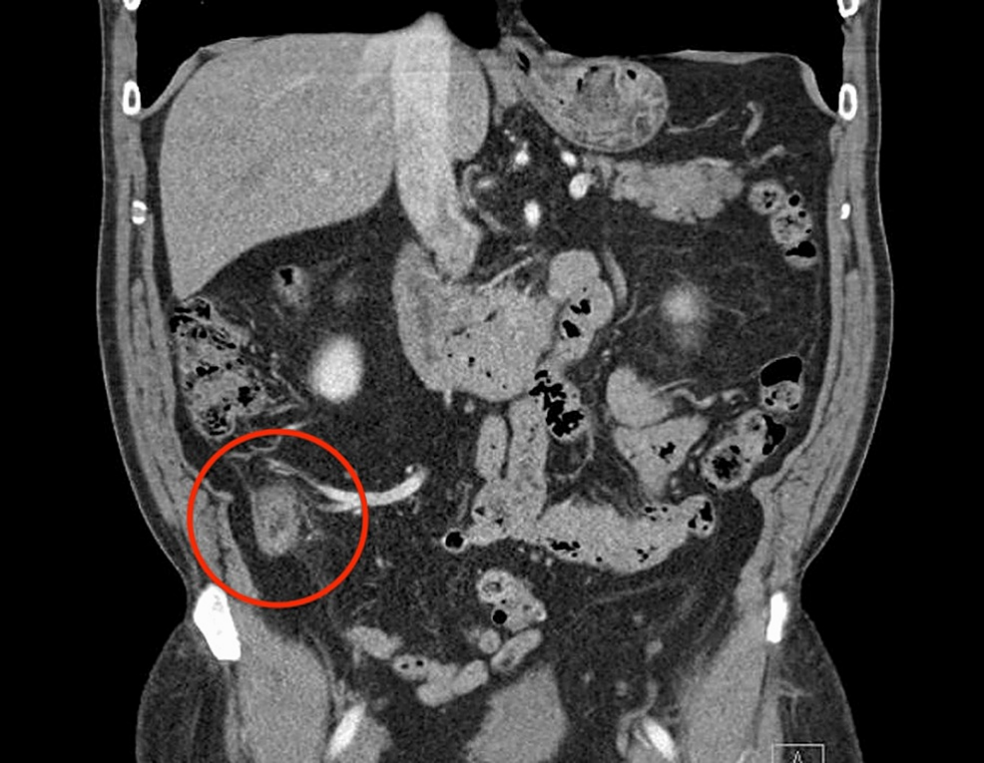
CT image (coronal View) showing appendiceal wall thickening.
